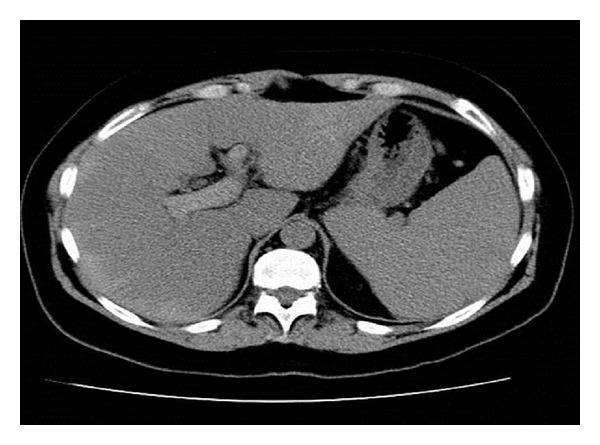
CT demonstrating portal thrombosis.
